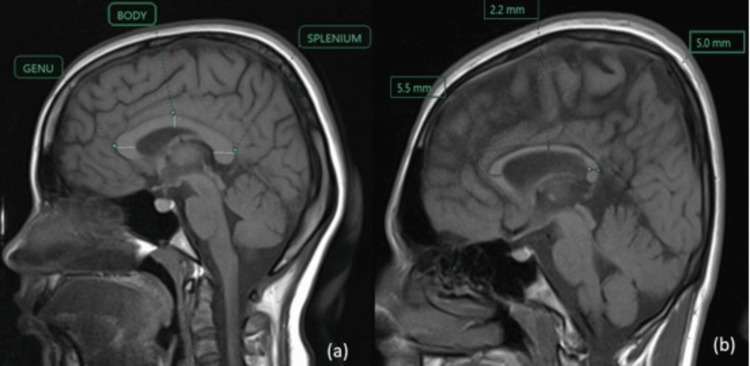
Mid Sagittal T1W magnetic resonance image Images show the measurement of the width of genu, body & splenium at their maximum level in control (a) and reduced width in case (b).
